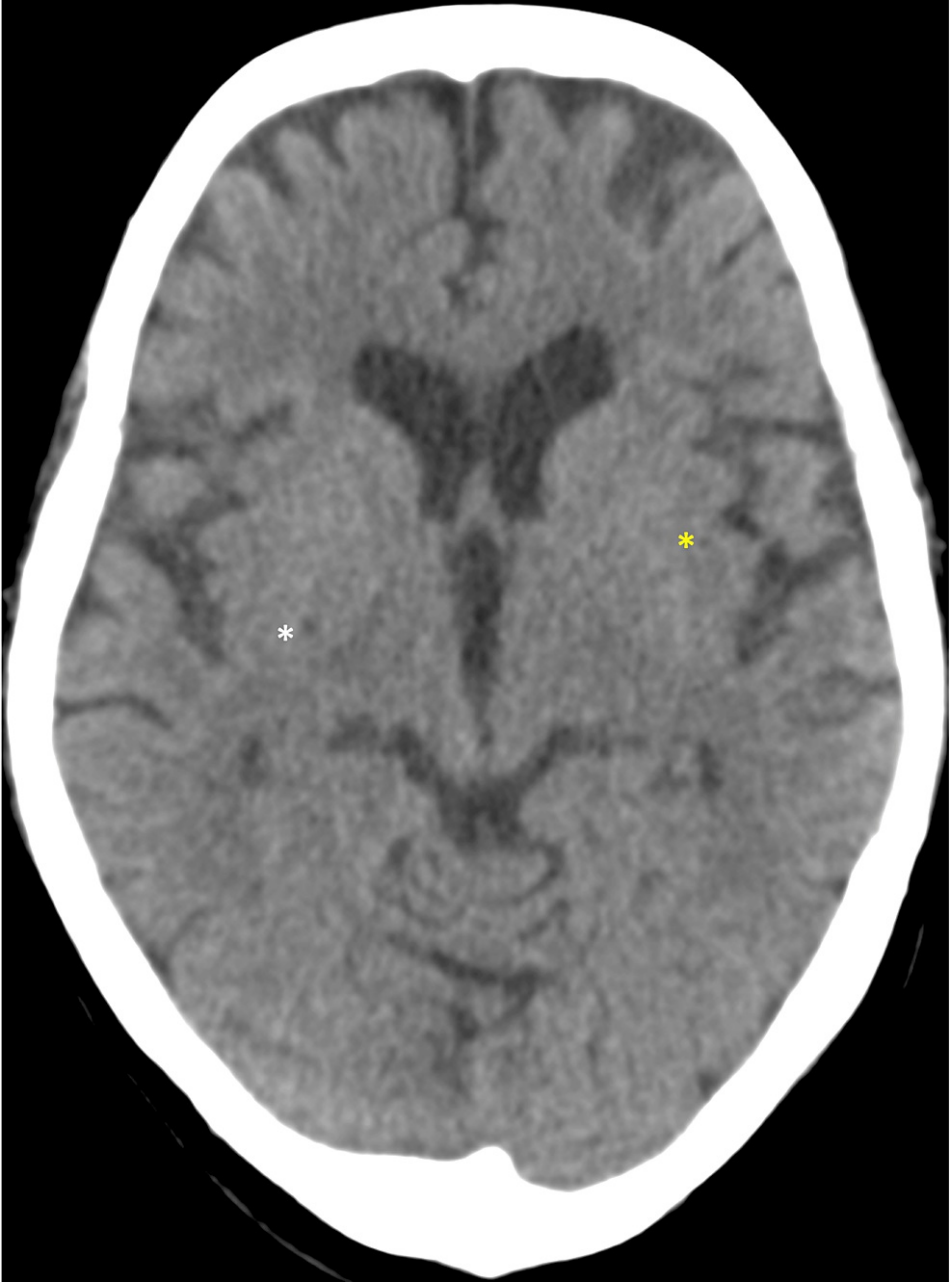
Contrast-enhanced computed tomography (CECT) of brain White asterisk (*) shows old lacunar infarcts in the right lentiform nucleus; yellow * shows old infarcts in the left external capsules.
